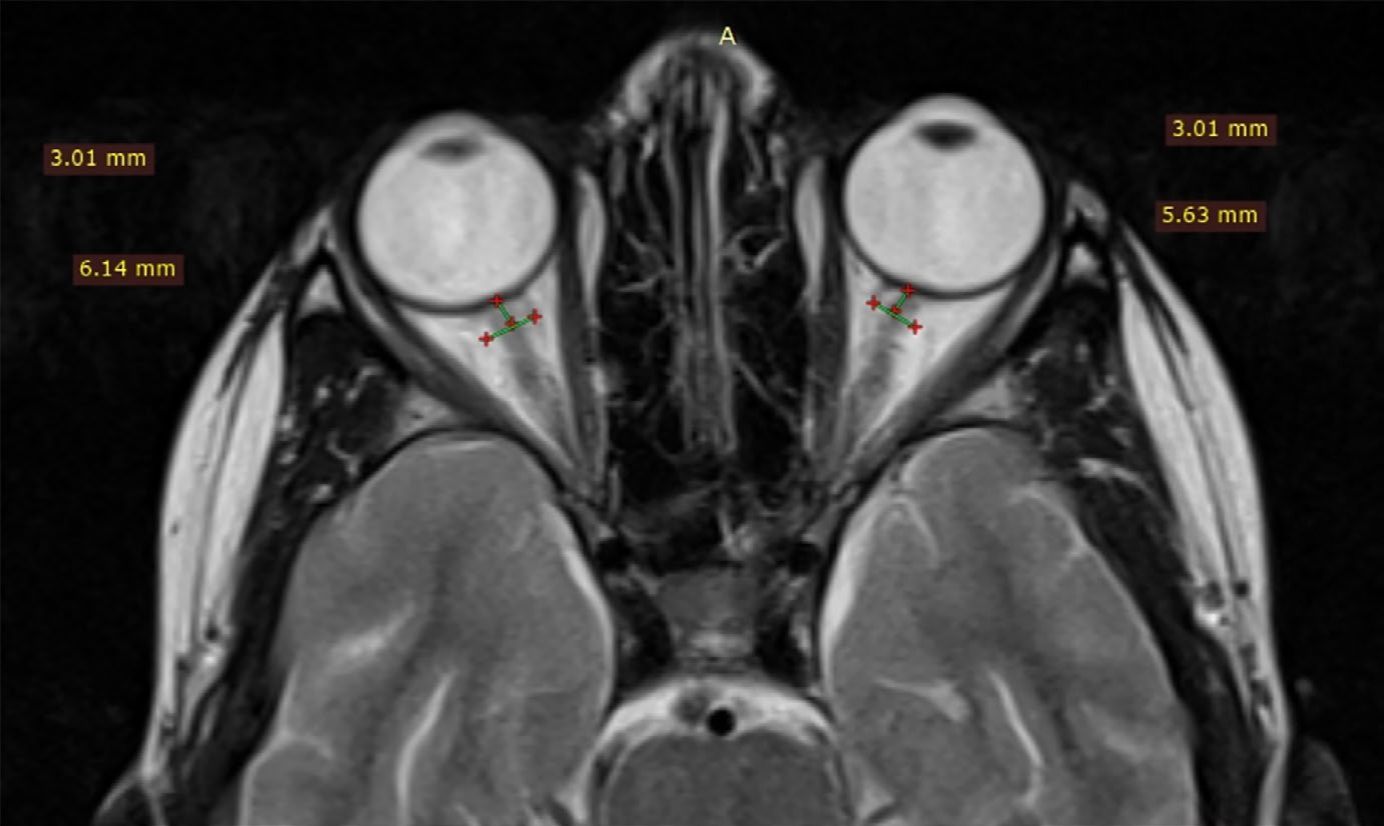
Brain magnetic resonance imaging showing prominent subarachnoid space around the optic nerves
